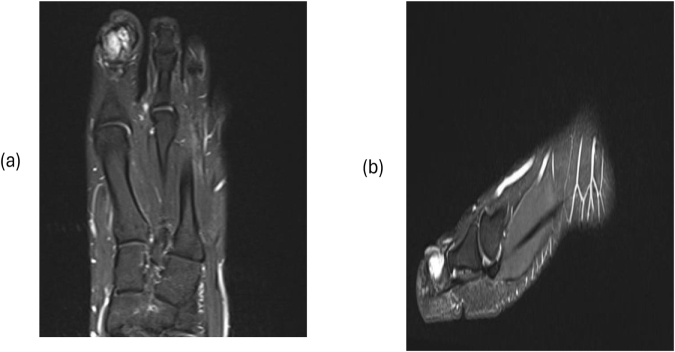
Anteroposterior X-ray of the left foot revealing an irregular and destructive lesion involving the distal phalanx of the first toe.
